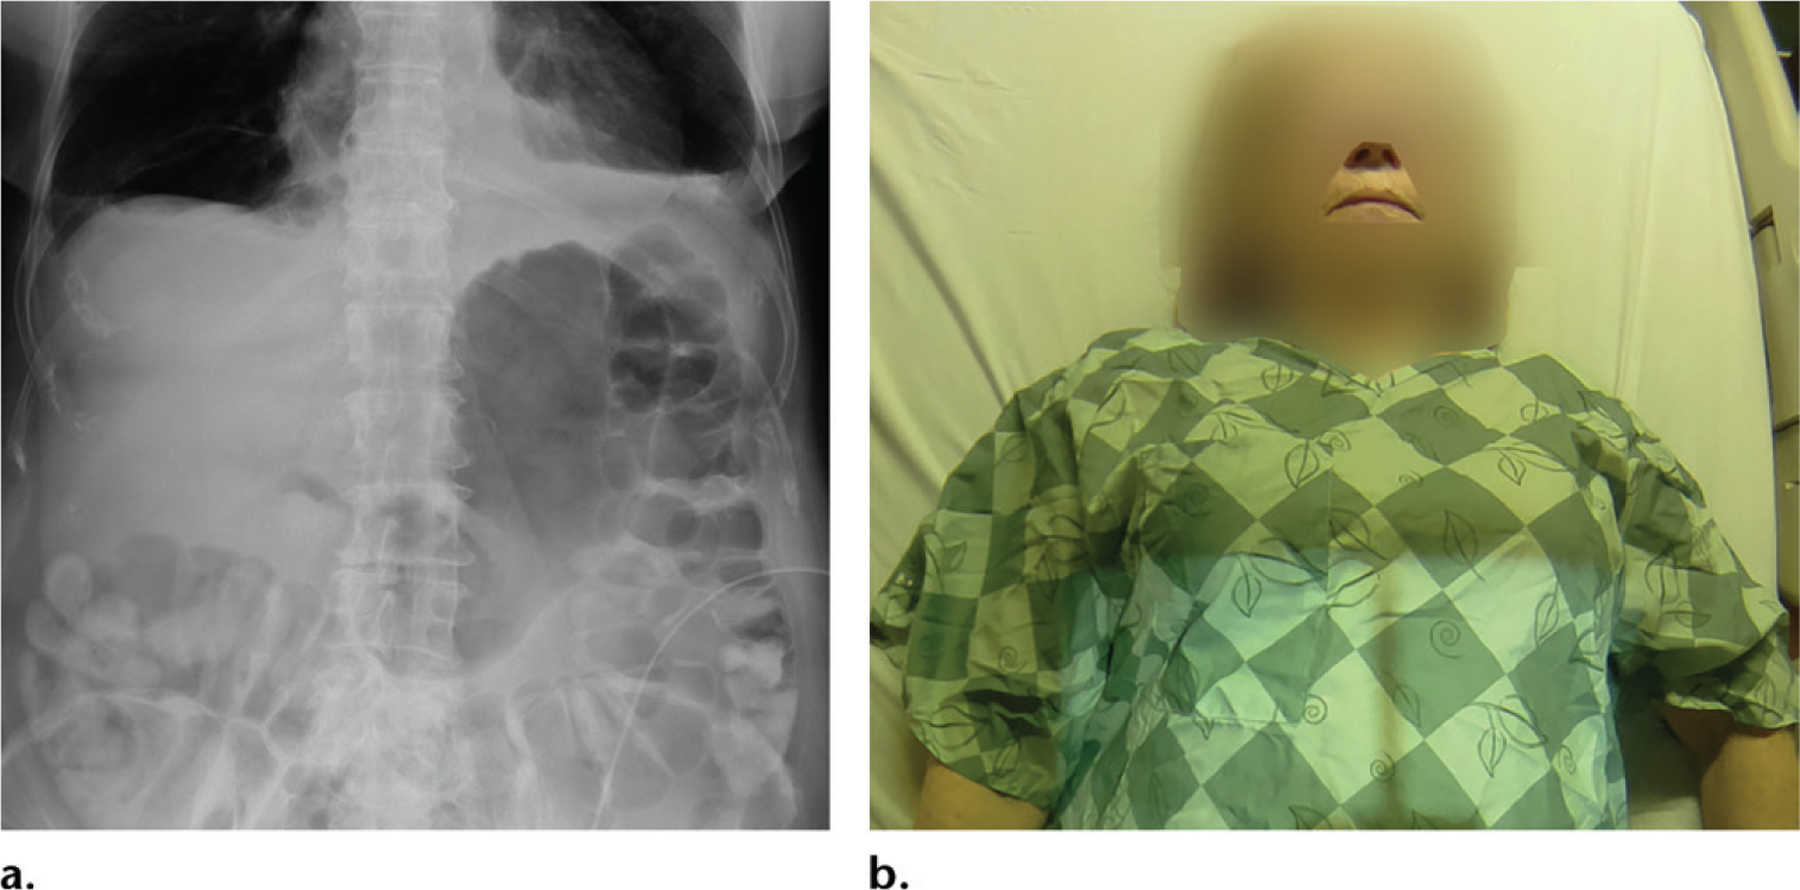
(a) Thoracoabdominal radiograph obtained to evaluate nasogastric tube position does not show an enteric tube in the lower chest or upper abdomen. The tube could potentially be coiled in the upper mediastinum or neck. (b) Photograph shows the patient with no tube entering the nares or the mouth. A call to the patient’s nurse or clinical service can be avoided with the review of point-of-care patient photographs.
